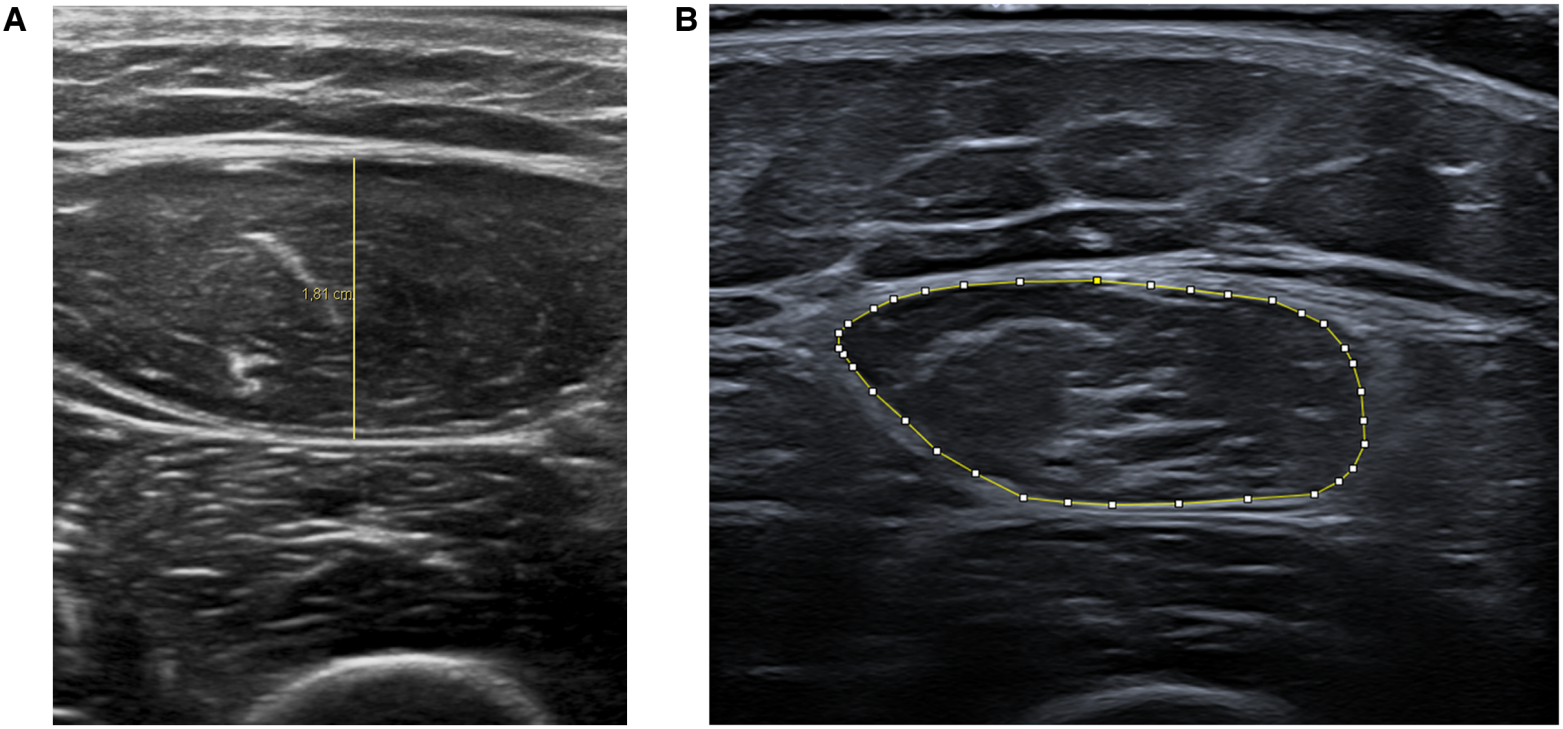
Representative ultrasound images showing rectus femoris muscle thickness (A) and cross-sectional area (B) measurements.
